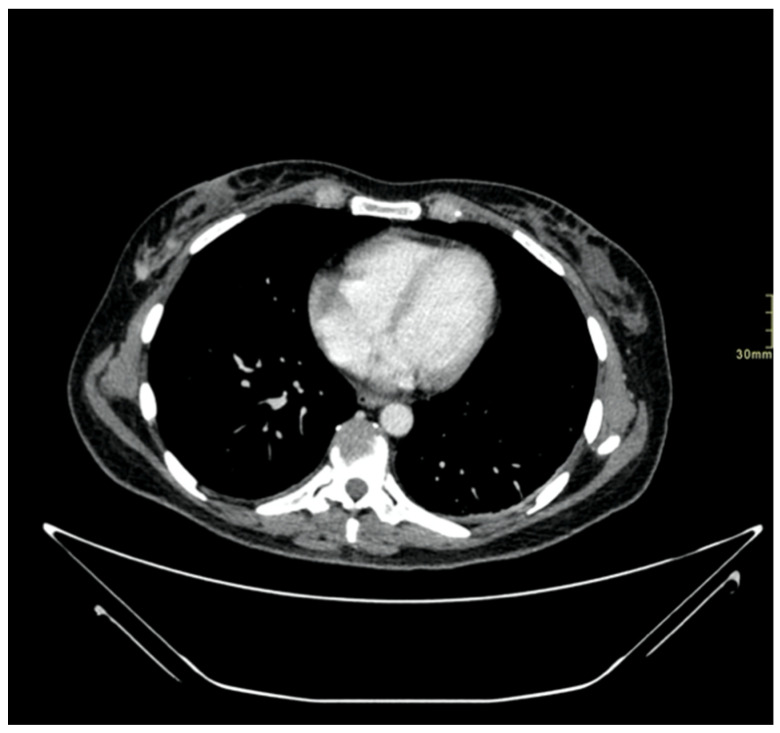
Multiple solid non-calcific nodules (at least 4 in the right QQEE), the largest measuring 13 × 9 mm revealed by the whole-body CT scan.
